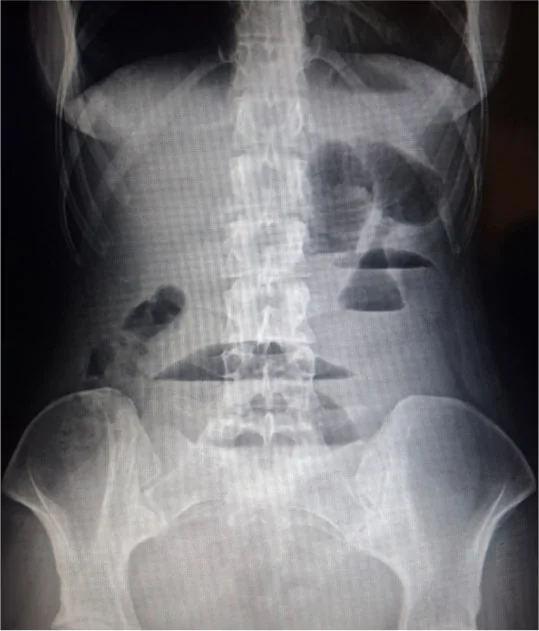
Plain abdominal radiograph showing centrally distributed air–fluid levels, wider than tall, consistent with small-bowel obstruction.
